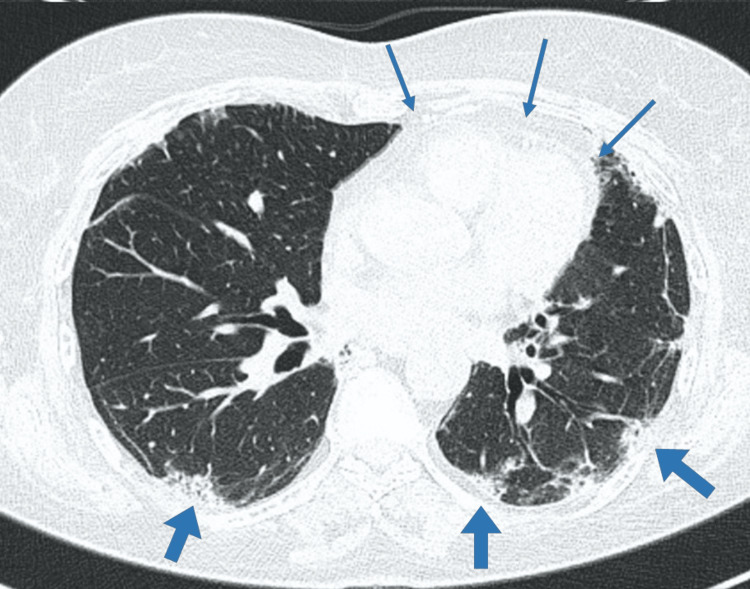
Re-evaluation chest CT scan No pneumomediastinum or subcutaneous emphysema were found (thin arrows). The previously described pulmonary chronic reticular interstitial pattern remained globally stable (thick arrows), now being interpreted as chronic fibrotic changes related to the underlying interstitial lung disease.
